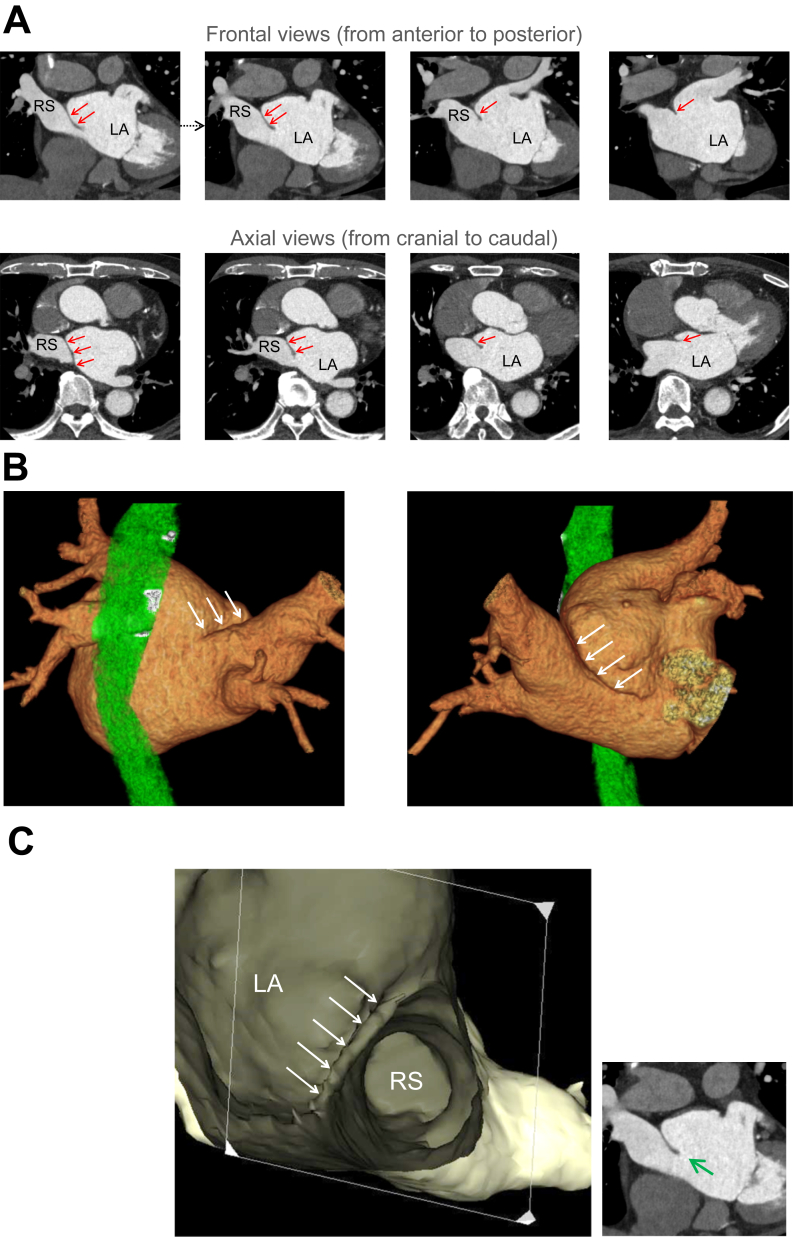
Frontal and axial computed tomographic views (A) and outer (B) and inner (C) 3-dimensional computed tomographic views showing an abnormal membrane (indicated by red or white arrows) dividing the left atrium (LA) into 2 chambers. In the inner view (C), the green arrow in the frontal view indicates the view direction. RS = right superior pulmonary vein.
